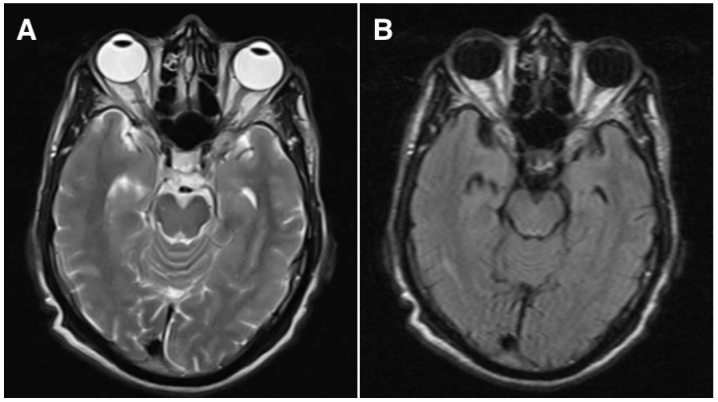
Magnetic resonance imaging of the brain and orbit by axial T2-weighted sequence (A) and fluid-attenuated recovery (FLAIR) sequence images (B) do not show any lesions in the choroid, optic nerve or orbit on both sides.
